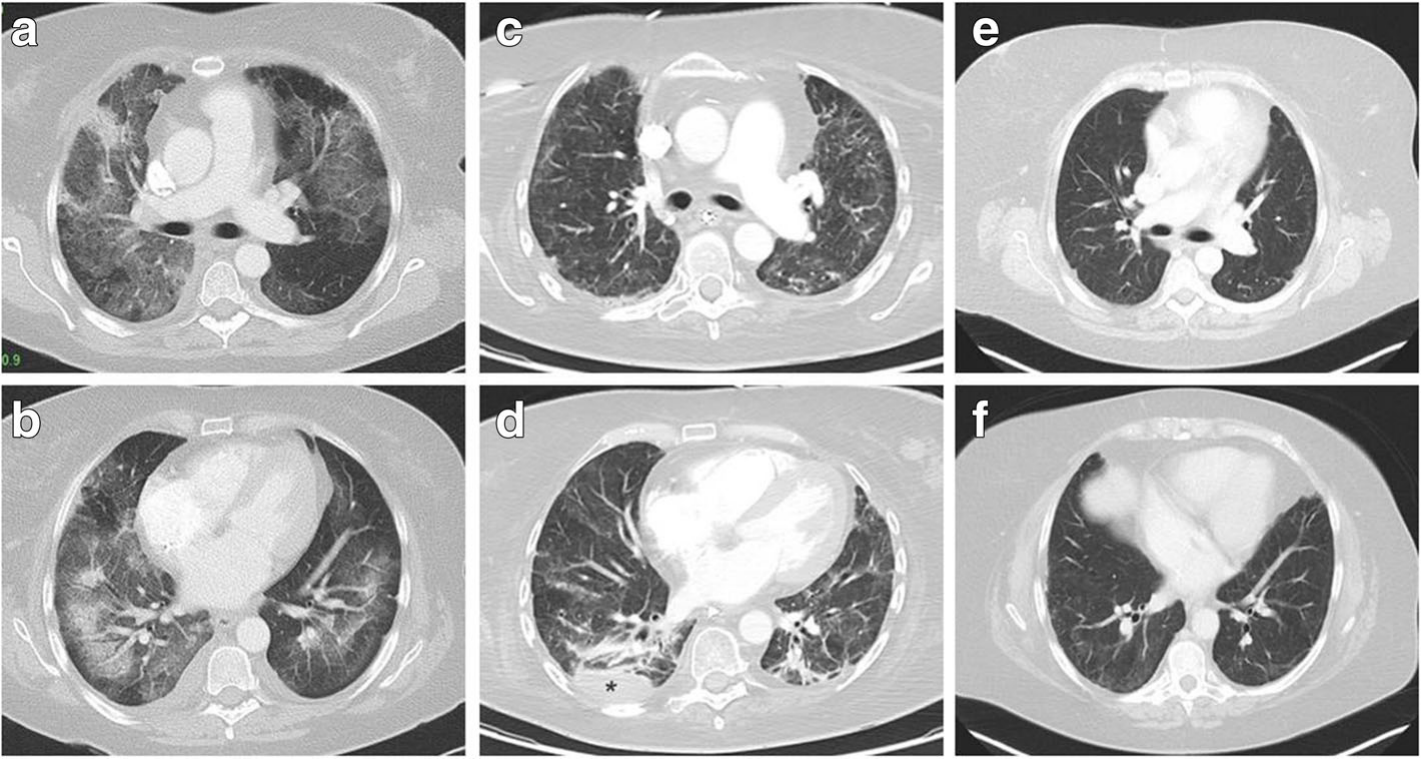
axial CT images of the chest in the lung window setting at the levels of the upper lobes (images in a, c and e) and the lower lobes (images in b, d and f). CT examination at the time of diagnosis revealed extensive abnormalities in both lungs with diffuse ground glass abnormalities in both the upper (a) and lower lobes (b). Associated areas of consolidation at the lower lobe level were present. Follow-up images 11 weeks after multimodal therapy for ILD revealed a good resolution of the ground glass abnormalities and consolidation with only minor parenchymal changes in the upper lobes, some small foci of ground glass abnormalities and some parenchymal bands (c). In the lower lobes, the nodular area (d asterisk) was consistent with loculated postoperative fluid due to the open-lung biopsy. The most recent examination more than 4 years after the event (e, f) showed no abnormalities
